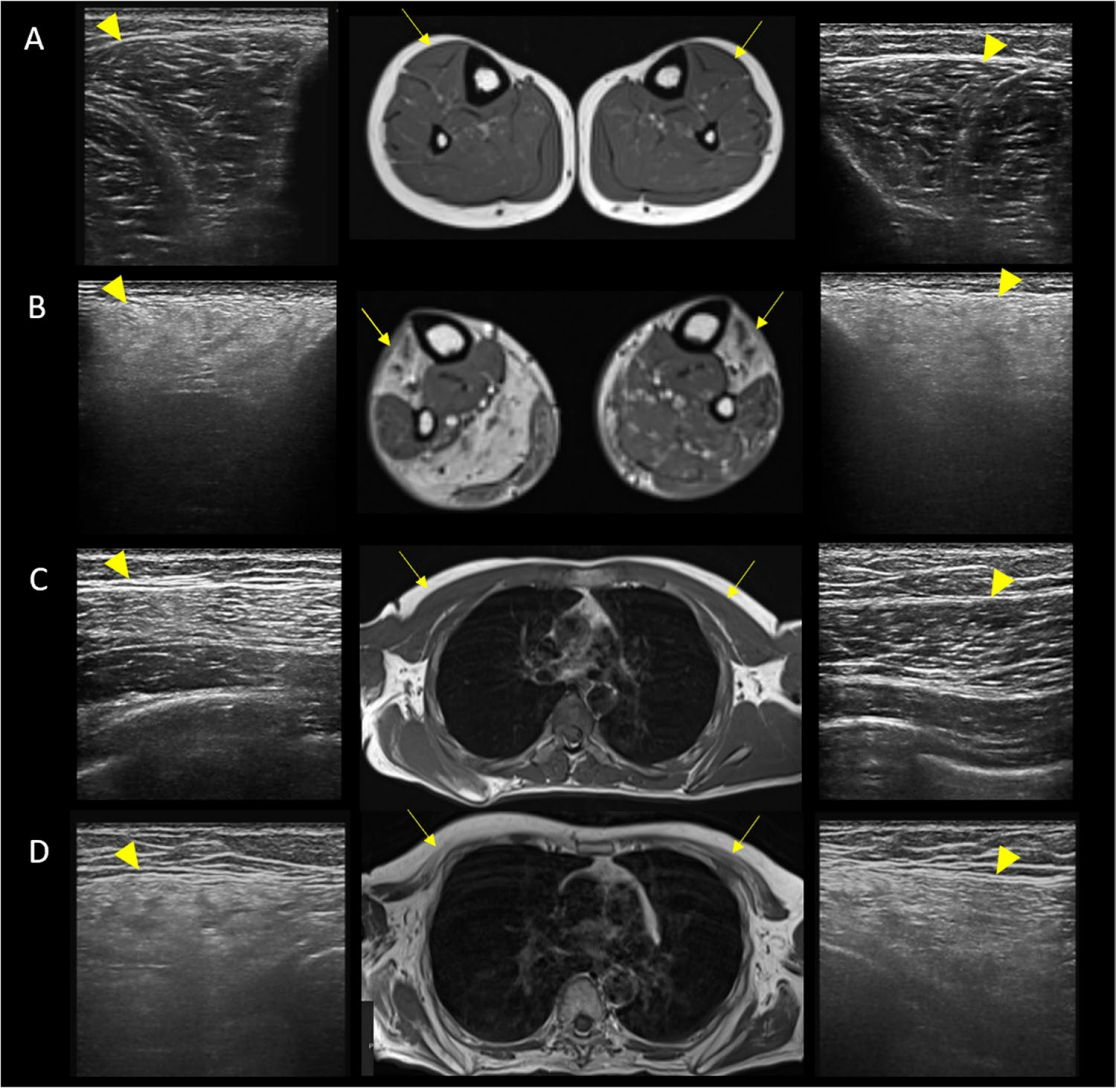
Muscle MRI and US matching. A Images of right and left tibialis anterior, appearing normal on MRI (Mercuri scale 0) (yellow arrows) and on US (yellow arrowheads). B Images of right and left tibialis anterior, completely fat replaced at MRI (Mercuri scale 4) (yellow arrows), and the corresponding US image that shows increased echogenicity and structural tissue changes (yellow arrowheads). C Images of right and left pectoralis major, appearing normal on MRI (Mercuri scale 0) (yellow arrows), while the right US image shows increased echogenicity and the left US image shows normal muscle echogenicity. D Images of right and left pectoralis major, appearing fat replaced at MRI (right: Mercuri scale 3, left: Mercuri scale 2) (yellow arrows), and the corresponding US image that shows increased echogenicity and structural tissue changes, more pronounced in the right muscle (yellow arrowheads)
